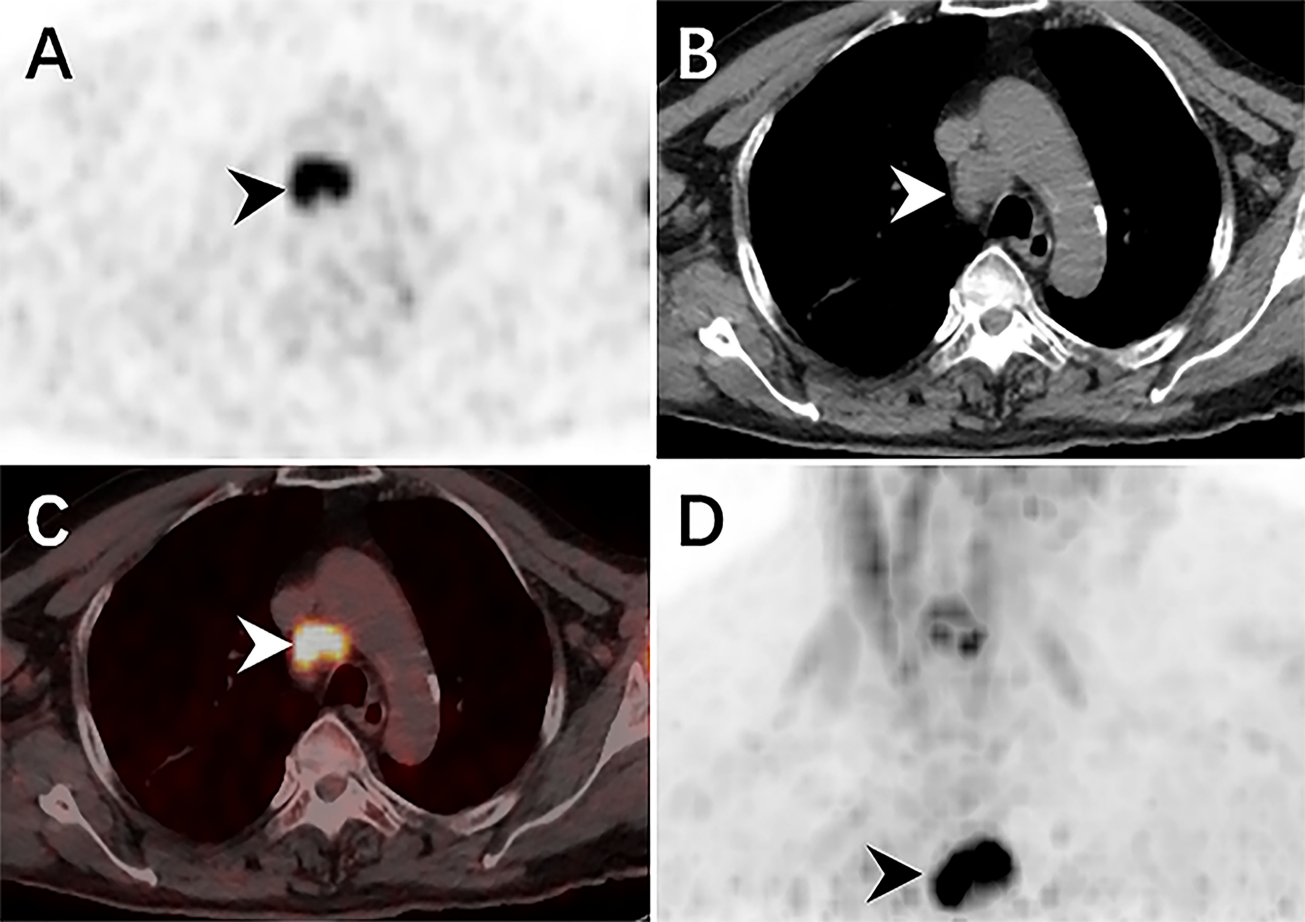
The delay ^18^F-FDG PET/CT revealed elevated activity foci in the mediastinum, with a SUVmax of 7.0. The CT scan revealed a single, low-density lymph node nearby the aortic arch (arrowheads) (A: PET, B: CT, C: fusion, D: MIP).
